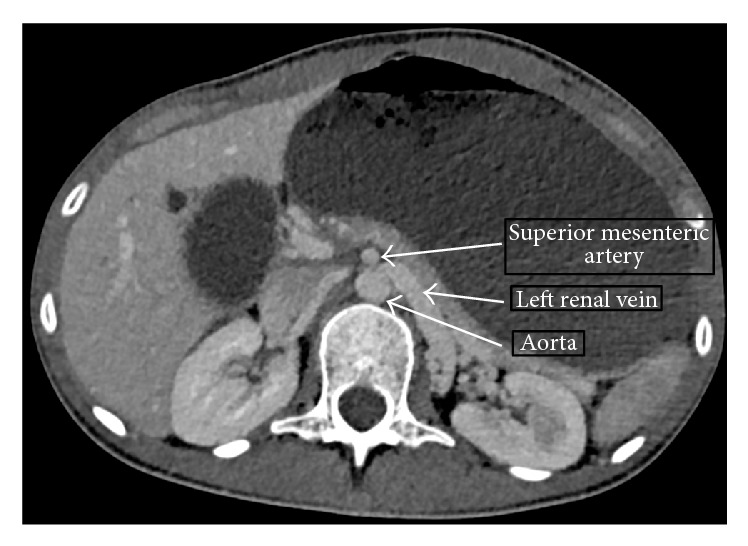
Axial CT section demonstrating the anatomy of Nutcracker phenomenon.
